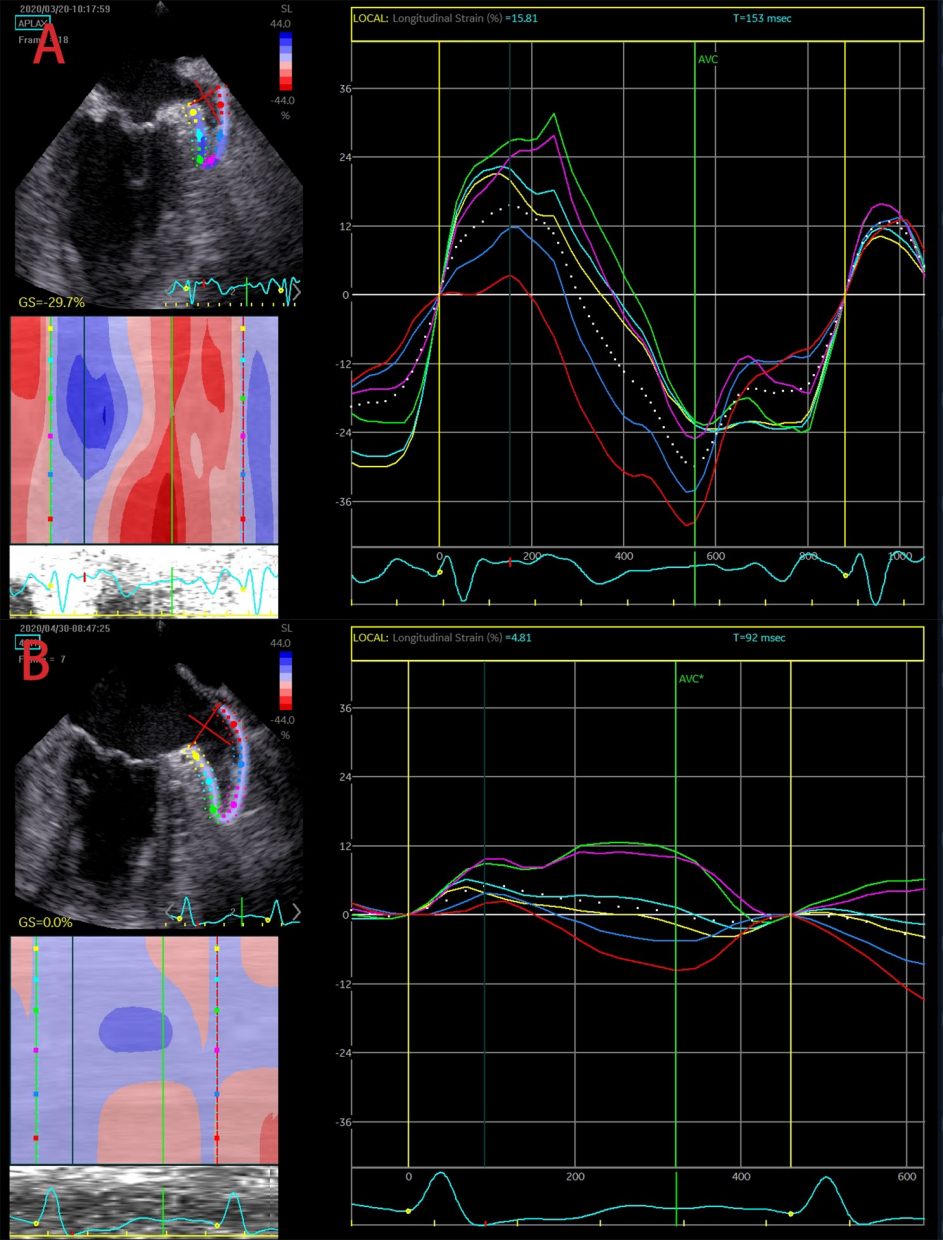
Echocardiographic speckle tracking imaging of LAA strain mapping in AF patients. (A) Nonrecurrence at 12 months after RFA; (B) recurrence at 12 months after RFA
